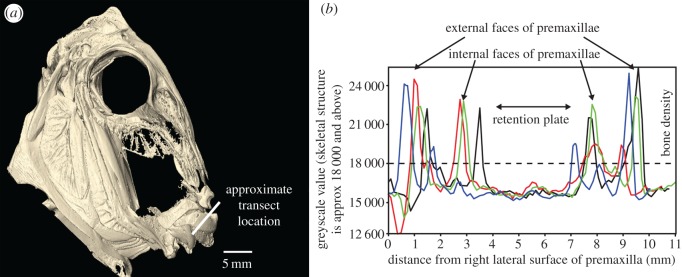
(*a*) A 3D model of the skull of *Ctenochaetus striatus*, generated using micro-computed tomography, showing where the transects were taken (electronic supplementary material, figure S5). (*b*) Four transects of greyscale data running from the right premaxilla towards the left premaxilla through the RP (the density of bone is conservatively estimated at 18 000) with densities across the RP matching that of surrounding soft tissues.
